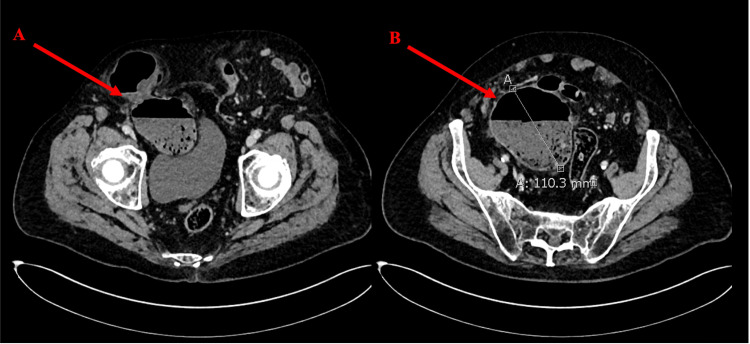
Contrast-enhanced computed tomography axial view of the pelvis. Large bilateral inguinal hernias are demonstrated. Label A demonstrates herniation of the distal descending colon and cecum into the hernia sac through a pinching direct defect. Label B demonstrates the cecum dilated to 11 cm with associated fecalization and a small amount of surrounding free fluid.
